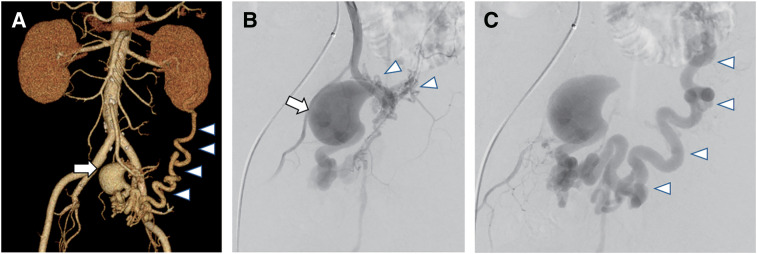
CT and angiography. (A) A contrast-enhanced CT shows an aneurysm-like dilatation of the IMAVF (arrow) and a tortuous drainage vein of the descending colon flowing into the splenic vein (arrowheads). (B, C) Selective angiography of the IMAVF shows a markedly dilated AVF (arrow) with a nidus supplied by multiple feeding arteries (arrowheads) originating from the inferior mesenteric artery in the early phase (B) and a drainage vein in the late phase (arrowheads) (C). AVF: arteriovenous fistula; CT: computed tomography; IMAVF: inferior mesenteric arteriovenous fistula
